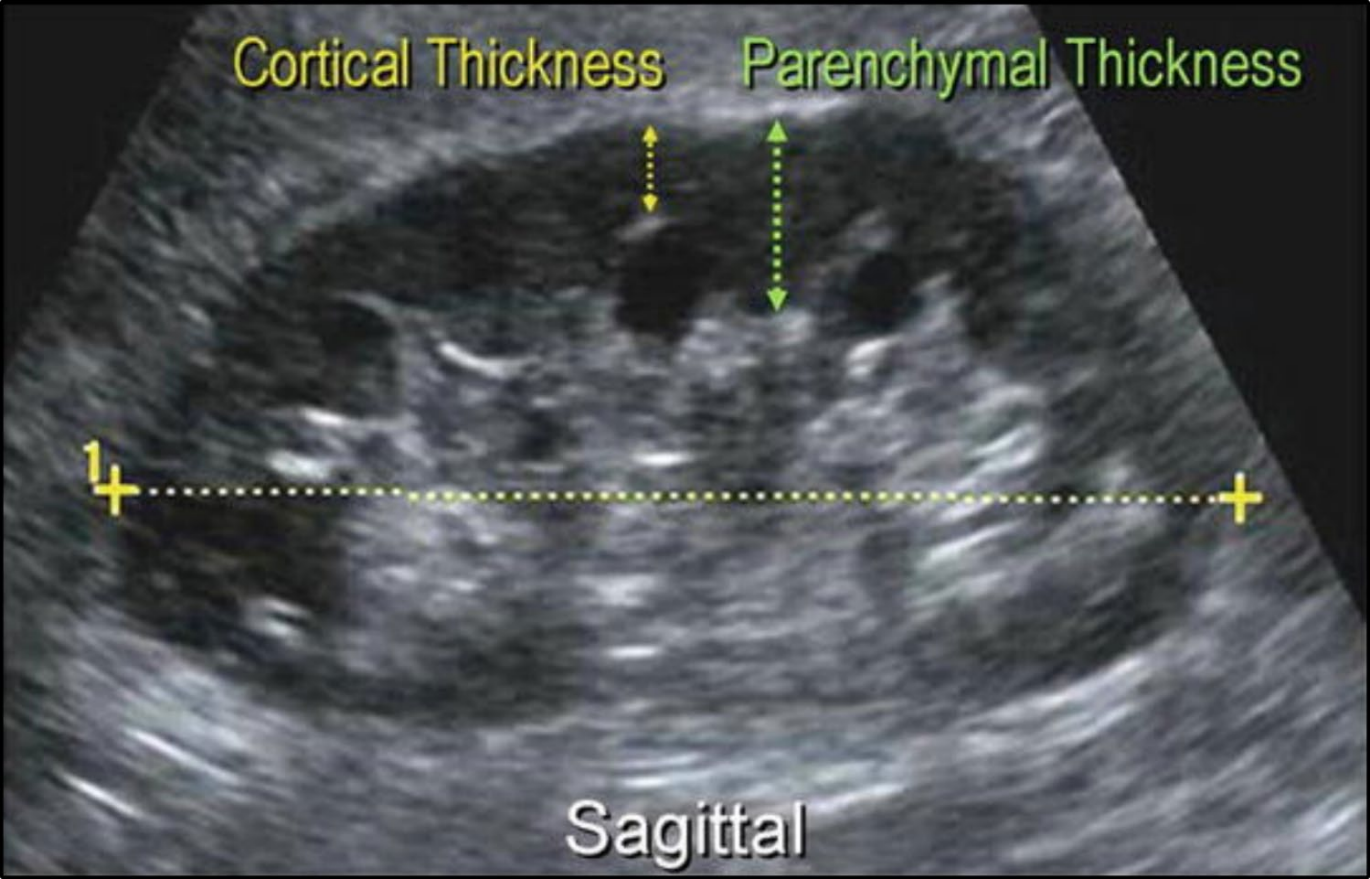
Measurement of renal cortical thickness, renal parenchymal thickness and kidney bipolar length by ultrasonography (Courtesy Campbell-Walsh urology 11th edition)[10]
